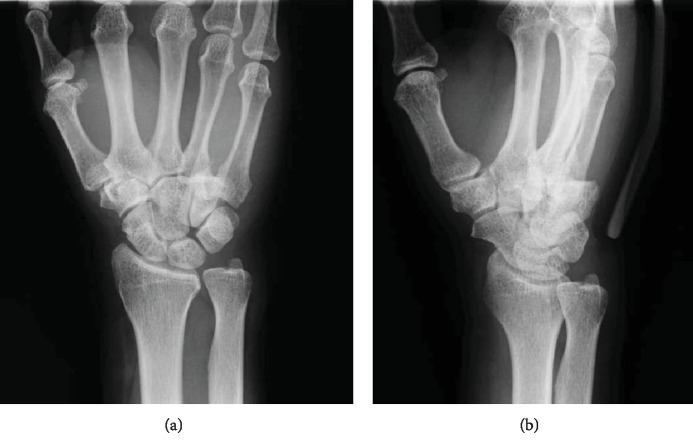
Initial radiograph showing the proximal displacement of the fourth and fifth metacarpal bones. (a) Posteroanterior view. (b) Oblique semisupinated view.
